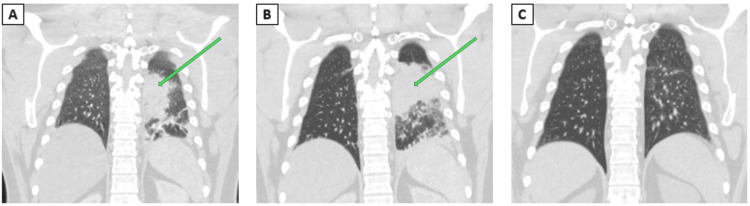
Chest CT of Case 1 (A) Left lower lobe airspace opacification on initial presentation marked by a green arrow. (B) Worsening left lower lobe opacification five days after initial presentation marked by a green arrow. (C) Resolution four months after the initial presentation CT: computed tomography
